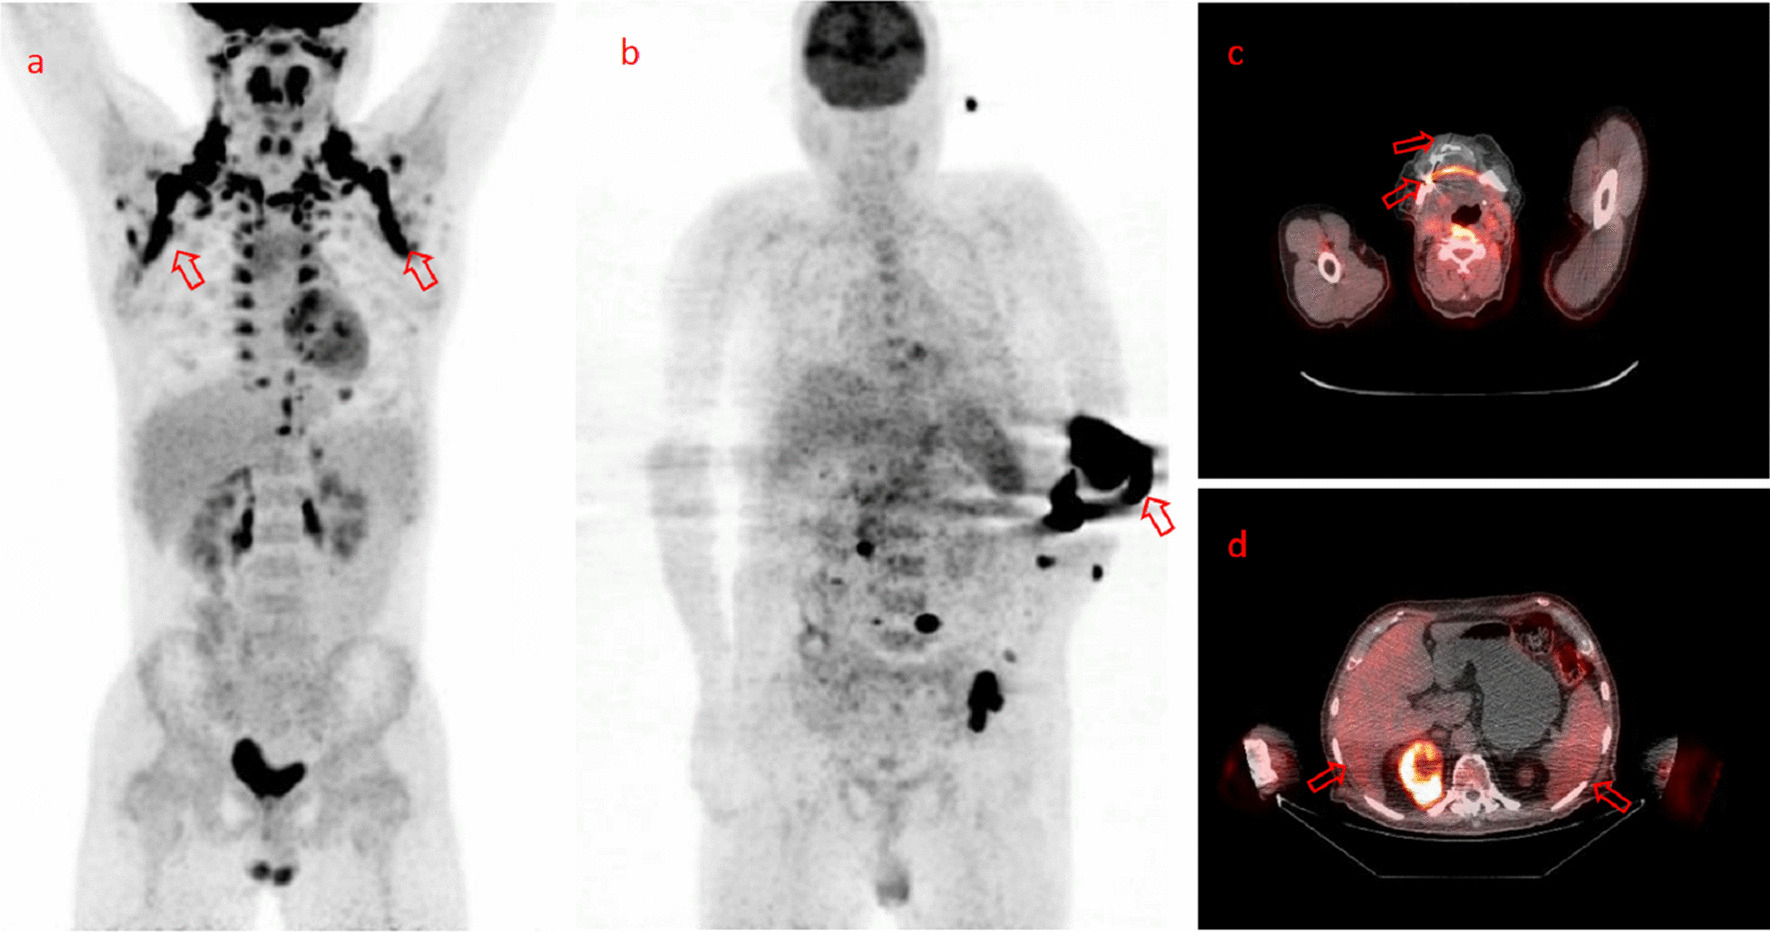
Typical ^18^F-FDG PET/CT images with insufficient level quality: a imaging of severe brown fat, b intravenous extravasation, c mismatch from external patient movement, d tissue over-attenuation from improper placement
